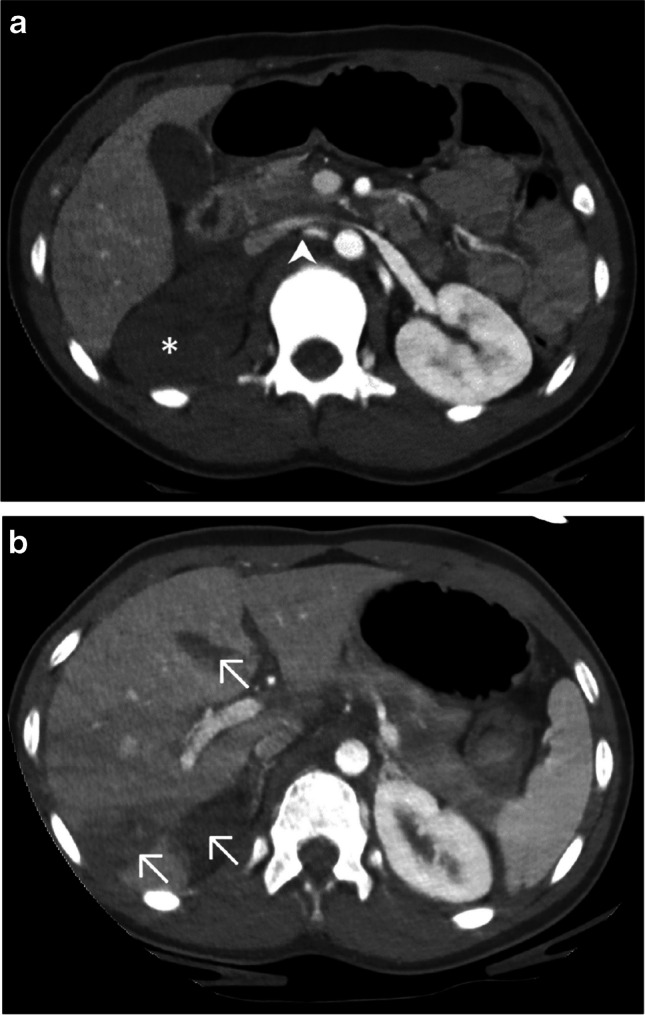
A 10-year-old girl with a history of a 20-m fall. Axial CT, post-contrast, following a split-bolus protocol, upper-abdominal images (soft tissue window). a Right renal infarction seen as a complete absence of enhancement of the kidney (*asterisk*) after renal artery dissection seen as a truncated arterial course (*arrowhead*). b American Association for the Surgery of Trauma (AAST) grade III hepatic lacerations seen as hypodense irregular stripes (*arrows*)
